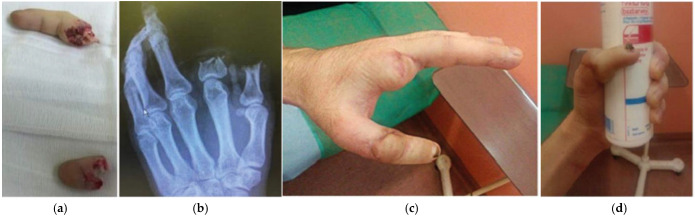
A case of a 24-year-old patient who had their thumb and index finger amputated. The thumb was not suitable for replantation due to the nature of the injury. It was therefore decided to replant the index finger in place of the lost thumb. (a) Presentation of the amputated fingers: the index finger (upper part of the figure) and the amputated thumb (lower part); (b) X-ray taken after the injury; (c,d) Final appearance after surgery, showing functional and aesthetic restoration five months postoperatively.
